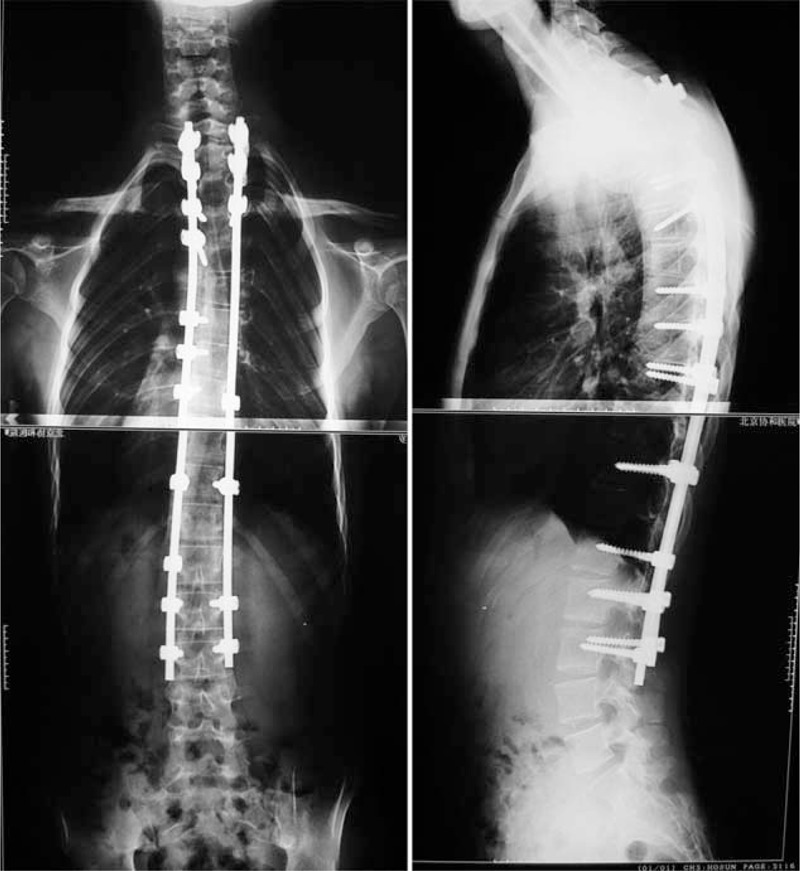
Standing anteroposterior and lateral radiographs of 48 months after operation.
